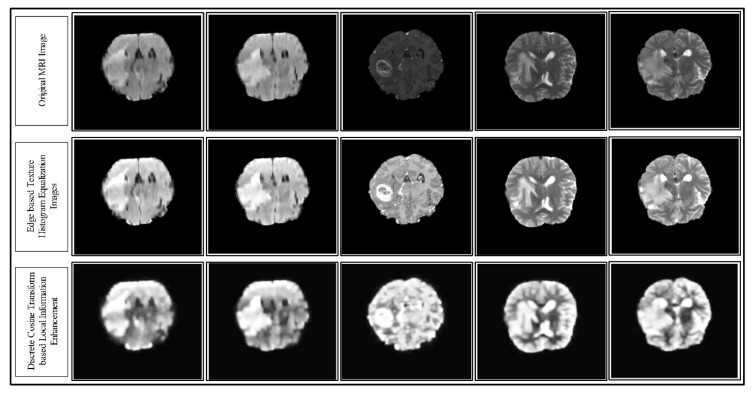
Results of the proposed hybrid local contrast enhancement using the MRI images of the Multimodal BRATS 2018 dataset. The results are shown from top to bottom.
